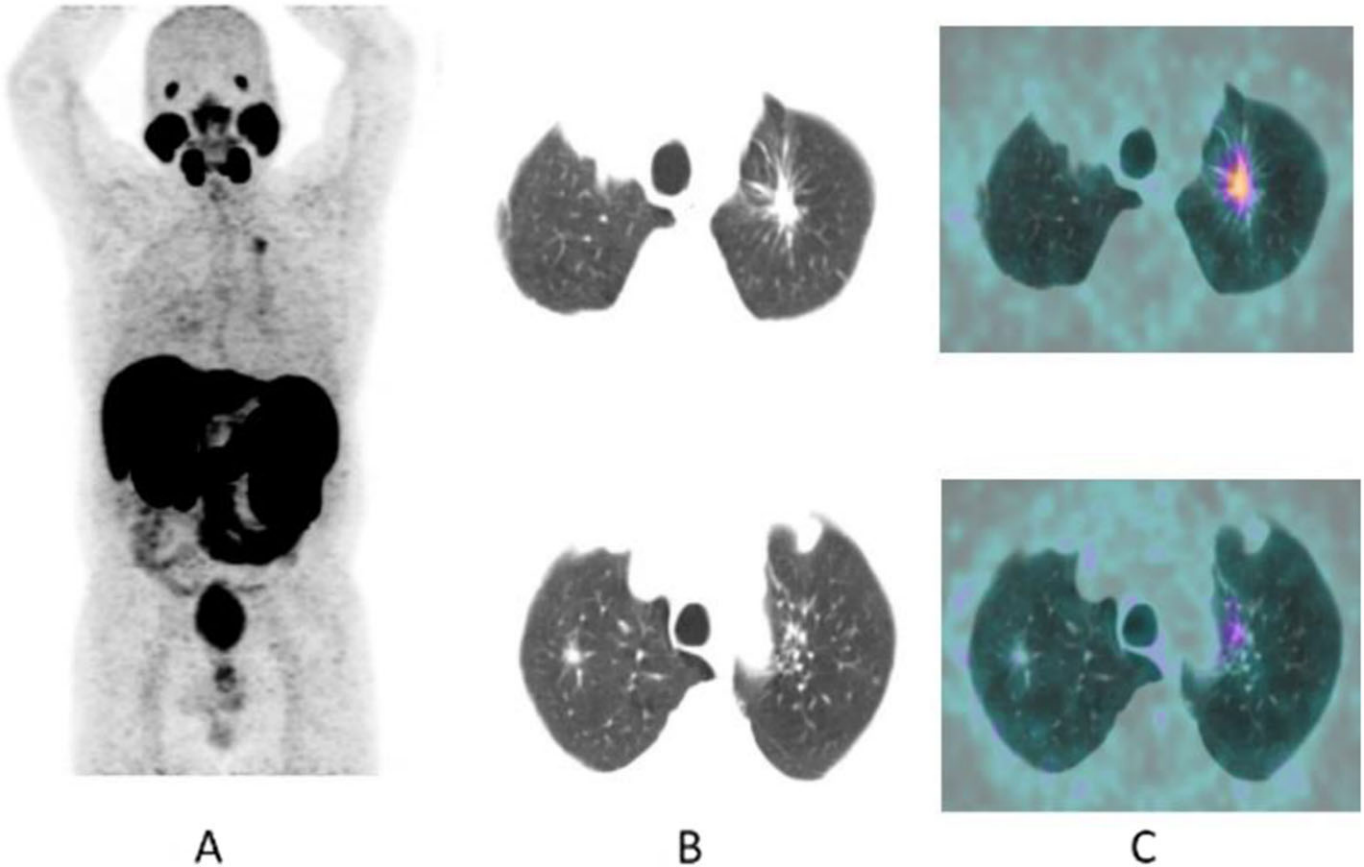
^68^Ga-PSMA, prostate cancer, initial staging: lung lesion. *Clinical history*: 68 y.o. patient diagnosed with prostate cancer, treated with radical prostatectomy. First biochemical recurrence: PSA 0.39 ng/ml. *PET/CT findings*: increased uptake in a pulmonary nodule with irregular margins and spiculated edges in the left upper lobe. Biopsy diagnosed a primary lung adenocarcinoma. a MIP, b CT images, c fused images
